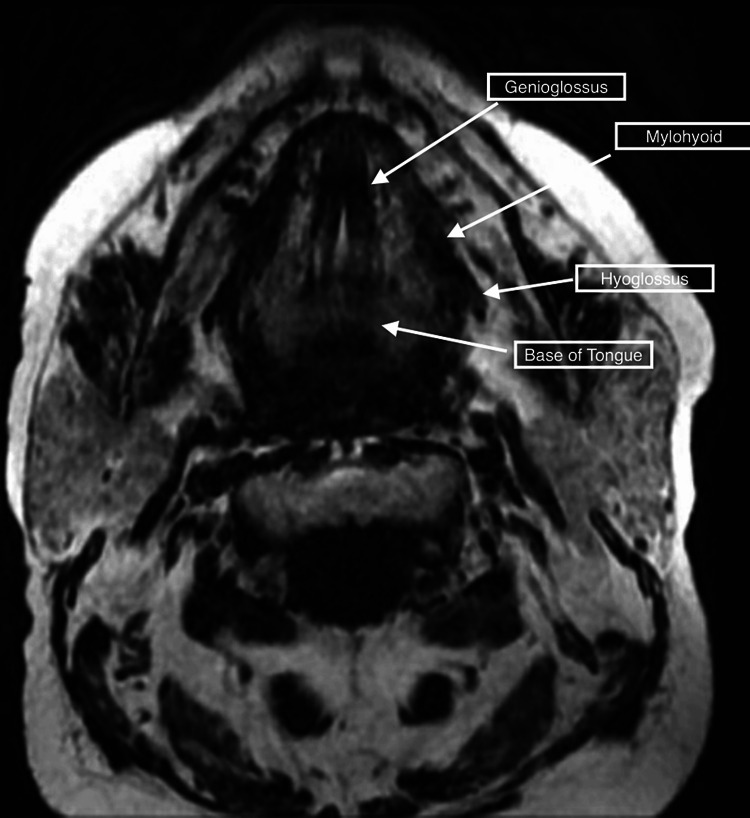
Axial facial MRI without contrast demonstrating masseter fatty infiltration. Tongue root and oral tongue musculature atrophy can also be seen.
